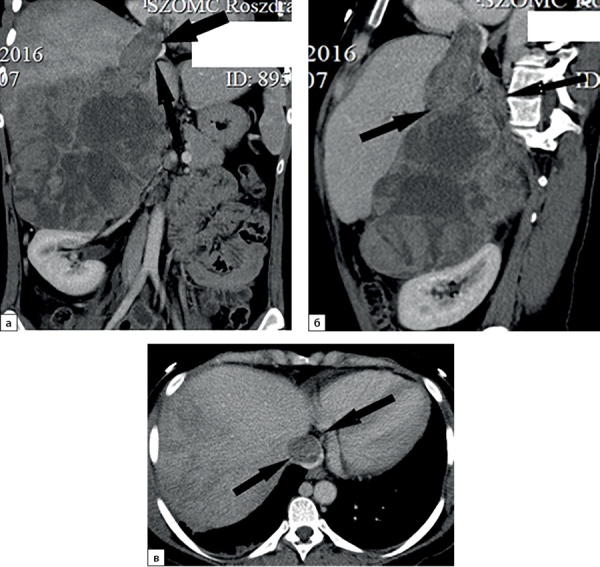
Рисунок 13. Компьютерные томограммы пациентки С., 24 лет, с гигантской опухолью правого надпочечника (165 мм), вызвавшей тромбоз нижней полой вены: а — изображение реформатированное в косой корональной плоскости, венозная фаза сканирования, опухолевый тромб в нижней полой вене (стрелки), пролабирующий в правое предсердие (стрелки); б — изображение реформатированное в косой сагиттальной плоскости, венозная фаза сканирования; в — венозная фаза, аксиальная плоскость.Figure 13. Computed tomography of patient S., 24 years old, with a giant tumor of the right adrenal gland (165 mm), which caused thrombosis of the inferior vena cava: a — image reformatted in an oblique coronal plane, venous phase of scanning, tumor thrombus in the inferior vena cava (arrows) , prolapsing into the right atrium (arrows); b — image reformatted in the oblique sagittal plane, venous phase of the scan; c — venous phase, axial plane.
